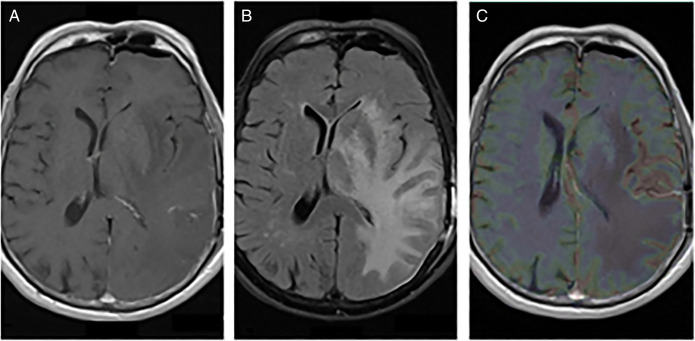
Postop imaging of L parietal lobe mass. (A) T1 axial 1 GD, (B) T2 FLAIR, and (C) T1 OLEA. FLAIR, fluid-attenuated inversion recovery.
